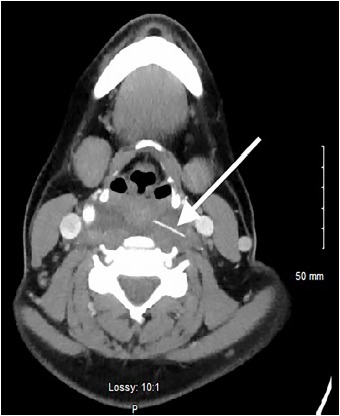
Computed tomography of the neck soft tissue with intravenous contrast in axial view, showing a linear metallic density with surrounding retropharyngeal effusion (white arrow).
